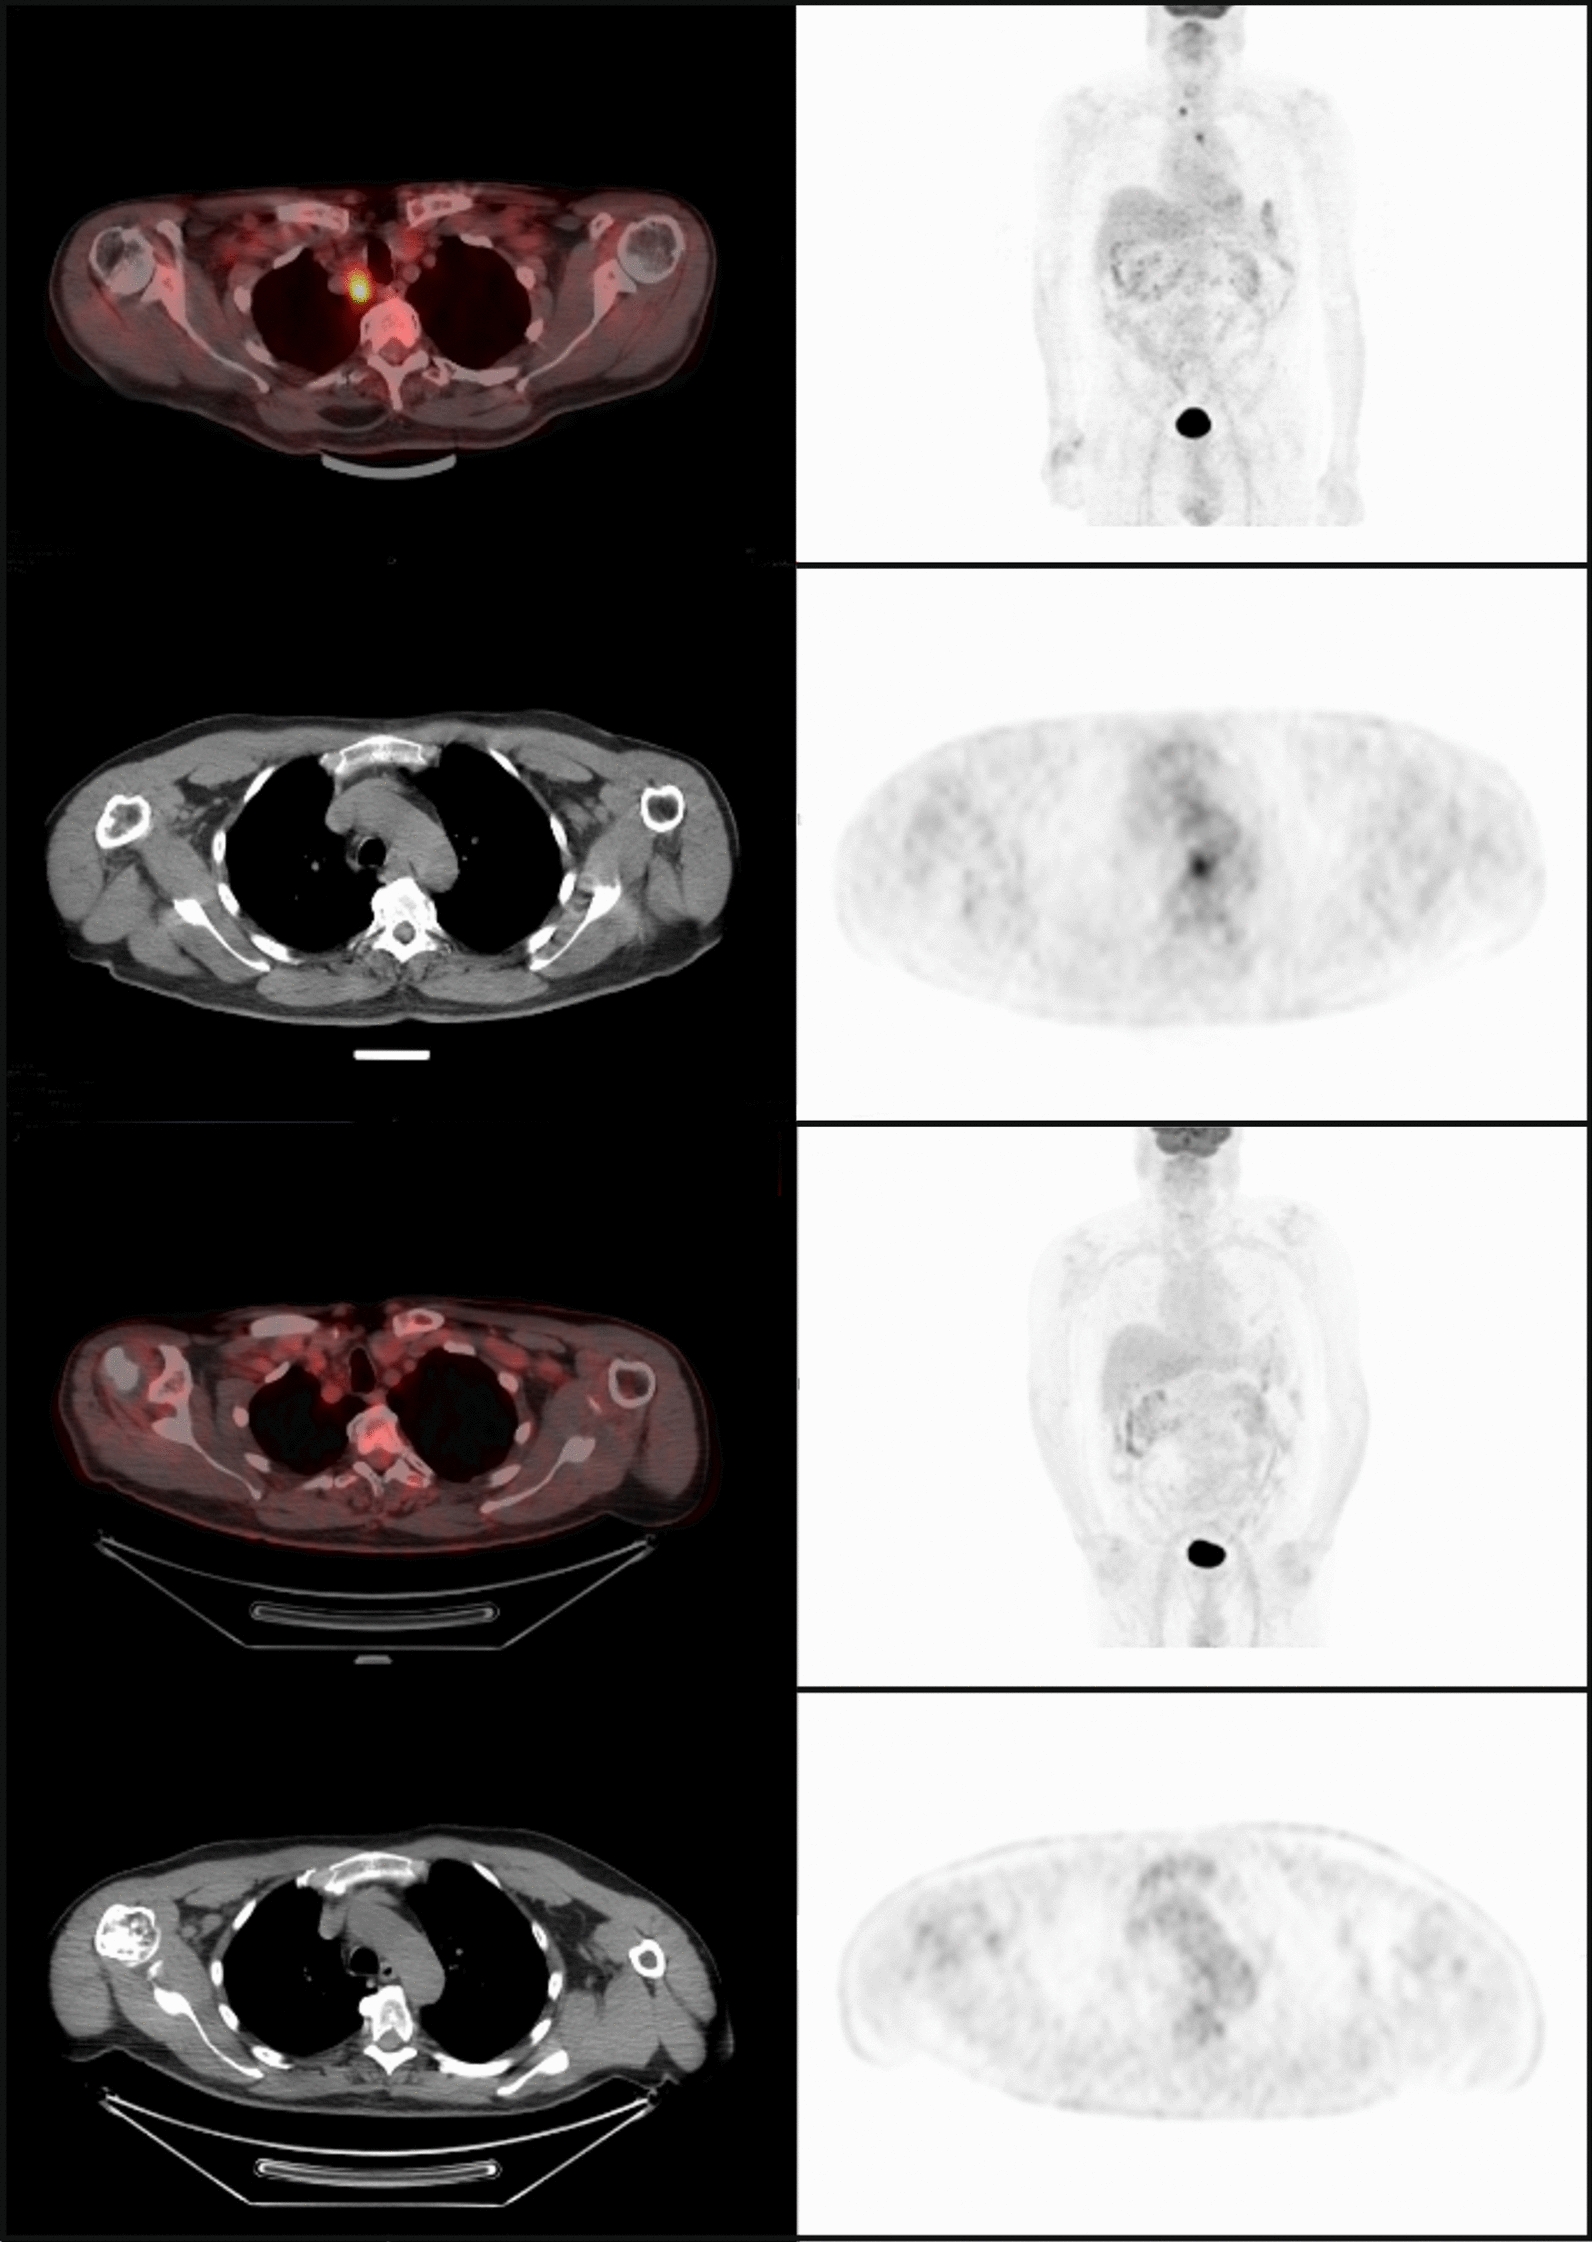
PET-CT scans of a patient with mMCC who achieved a complete response with avelumab. Images were provided by Dr. Carnaghi. *mMCC* metastatic Merkel cell carcinoma, *PET-CT* positron emission tomography–computed tomography
